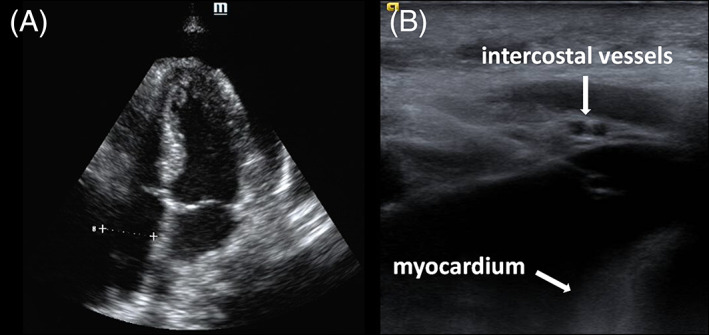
Moderate symptomatic MPE in a 46‐year‐old woman with a history of breast cancer. (A) Conventional echocardiogram performed before the procedure showed a moderate pericardial effusion. The maximal anterior depth was 19 mm. (B) The needle is continuously visualized by a high frequency ultrasound while entering the pericardial space. A safe approach to needle advancement under high frequency ultrasound guidance while continuous visualization of the needle insertion, superficial part of the pericardium, intercostal vessels and myocardium
